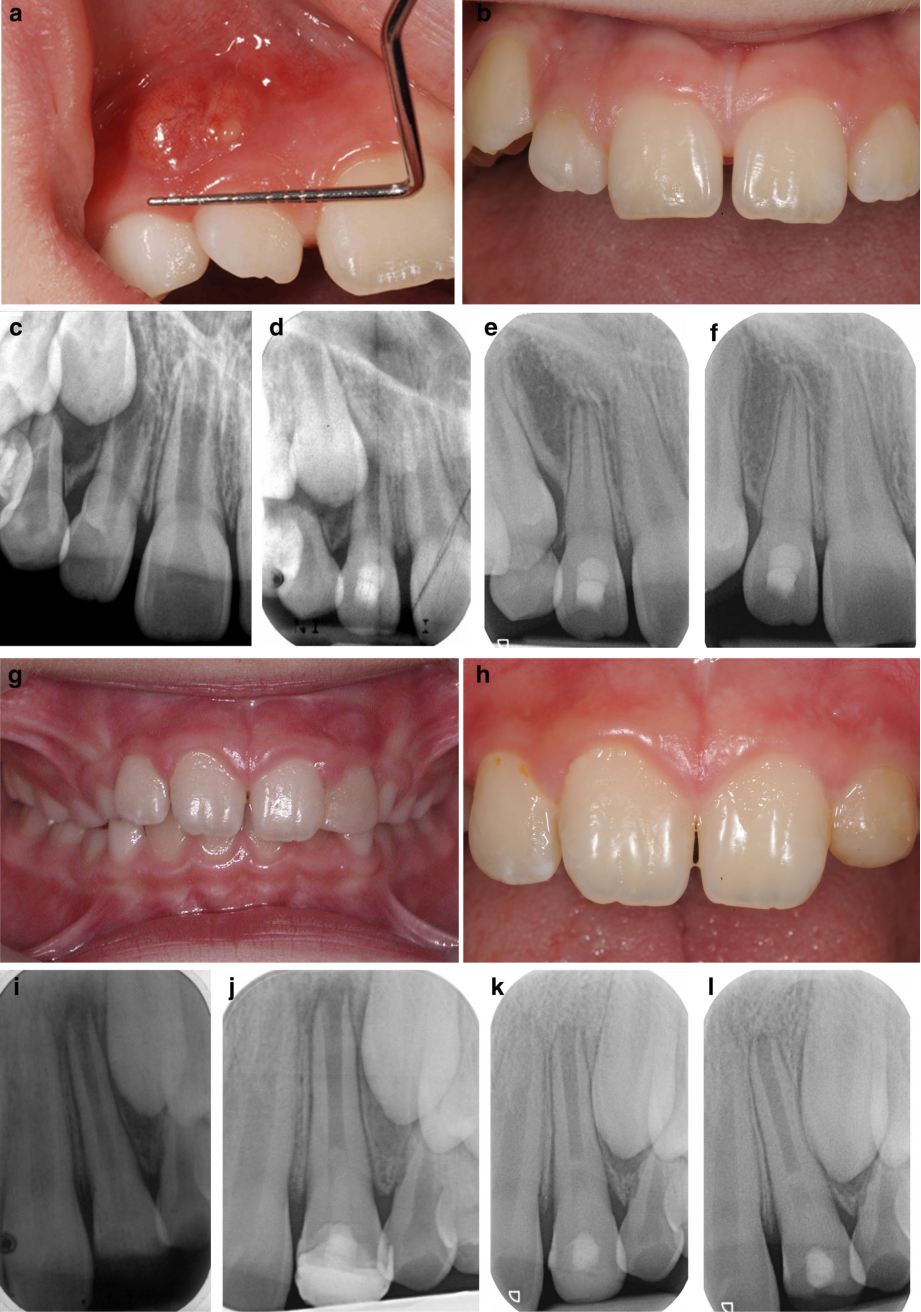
Clinical photographs and radiographic examination of two cases treated with regenerative endodontic technique showing success and survival outcomes. a Photograph showing labial abscess with discharging sinus related to the non-vital 12 secondary to dens invaginatus. b Photograph showing resolution of signs of infection (swelling and discharging sinus) maintained for up to 24 months following RET treatment of 12. c–f Periapical radiographs taken at baseline (showing an immature 12 with <1/2 root formation, thin dentinal walls and wide open apex), and follow-up at 3 months, 9 months and 2 years showing complete success following RET with gradual root formation and thickening of dentinal root walls. g Photograph showing traumatised non-vital 21 which sustained an enamel/dentine fracture. h Photograph showing no signs of infection (swelling and/or discharging sinus) at 24 months following RET treatment of 21. i–l Periapical radiographs taken at baseline (showing an immature 21 with <2/3 root formation, thin dentinal walls and wide open apex), and follow-up at 3 months, 9 months and 2 years showing no evidence of periapical lesion and with no signs of continuation of root development nor thickening of dentinal walls. The apical root canal space (l) shows evidence of radiopaque trabeculation suggestive of bony ingrowth
